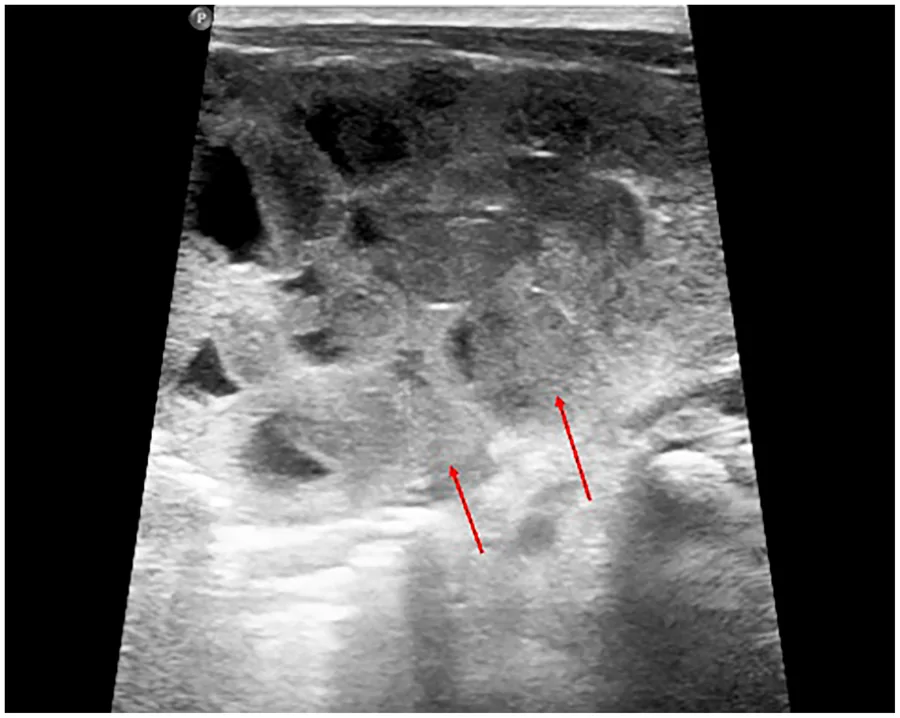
Ultrasonography in a late preterm, low-birth-weight male infant with culture-proven candidemia demonstrates bilateral renomegaly with increased renal echogenicity. Multiple well-defined hypoechoic lesions in the right renal parenchyma are consistent with renal microabscesses. Linear echogenic foci within both pelvicalyceal systems are suggestive of fungal balls. The arrows indicate the renal microabscesses.
